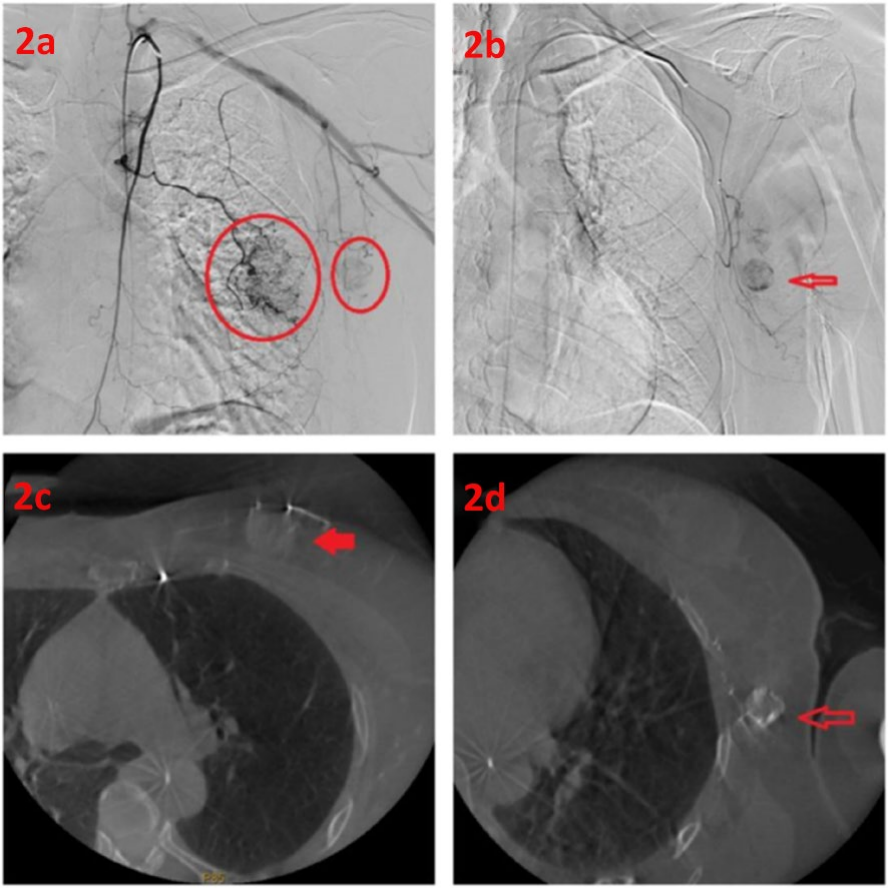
Intraoperative contrast imaging and CBCT imaging. (a), (b) Angiography of breast tumors and axillary metastatic lymph nodes was performed using microcatheter superselective intubation. (c), (d) CBCT scanning can visualize breast tumors and axillary lymph node metastasis.
